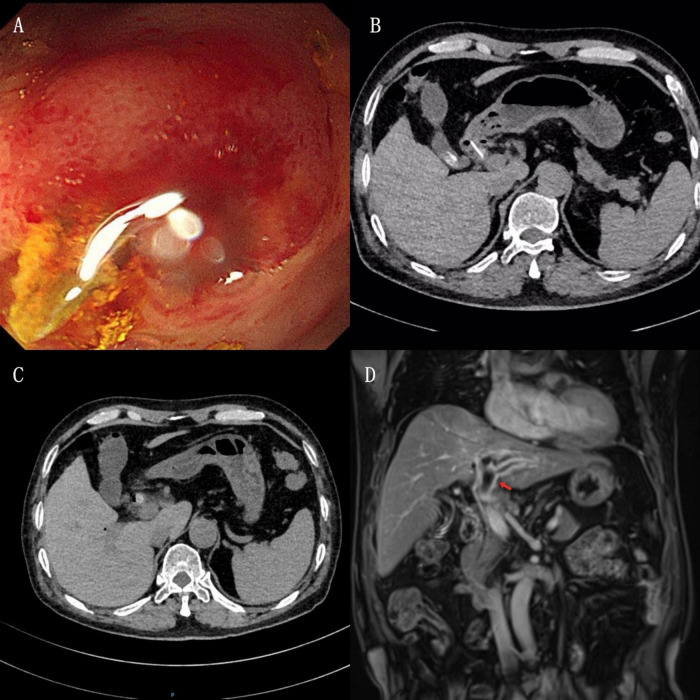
(A) Gastroscopy shows a sharp white foreign body in the duodenal bulb, partially embedded in the intestinal wall. (B) CT plain scan reveals a high-density shadow in the duodenum, closely associated with the intestinal wall. High-density shadows are visible in the gallbladder, consistent with gallstones. (C) CT plain scan shows a high-density shadow in the duodenum, slightly smaller than before. Common bile duct stones are observed, with a suspected duodenal foreign body extending into the common bile duct. (D) Contrast-enhanced MRI shows thrombus formation in the left branch of the portal vein (indicated by the red arrow).
